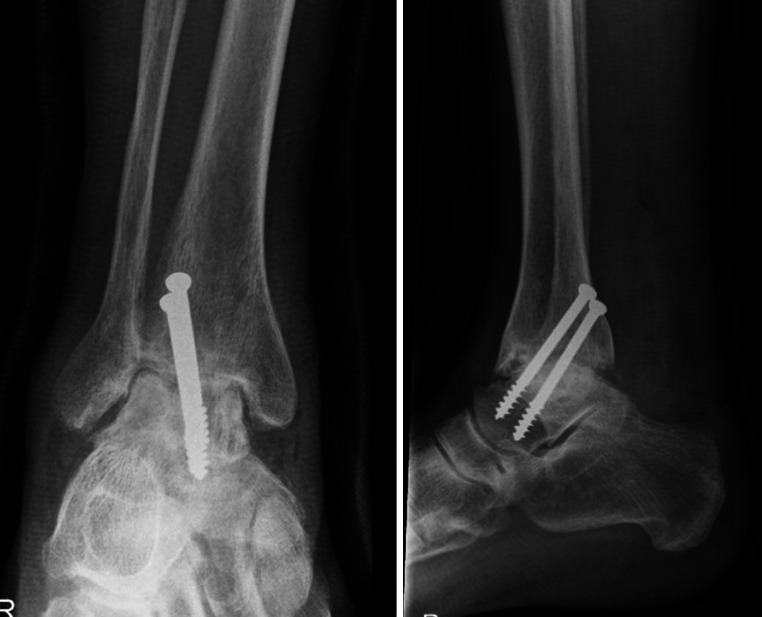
Radiographs in the anterior-to-posterior and lateral direction at 2 weeks following posterior arthroscopic ankle fusion with the two screws in an orientation from posterolateral to anteromedial over the ankle joint
